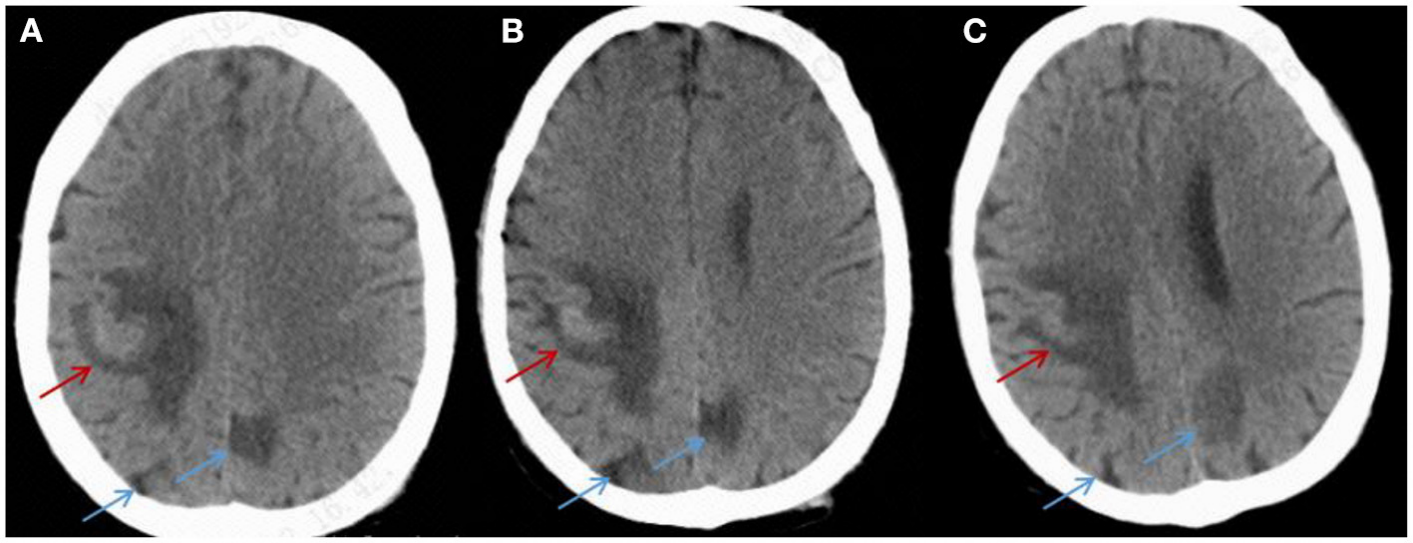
CT imaging for the patient's brain. (A) On day 25, a CT scan of the brain showed hyperdense lesions with surrounding edema in the right parietal lobe (red arrow) and hypodense areas (blue arrow) in the left and right parietal lobes. (B,C) After 2 and 3 weeks of treatment, a CT scan showed that the lesions were absorbed in the left and right brain (red arrow and blue arrow).
